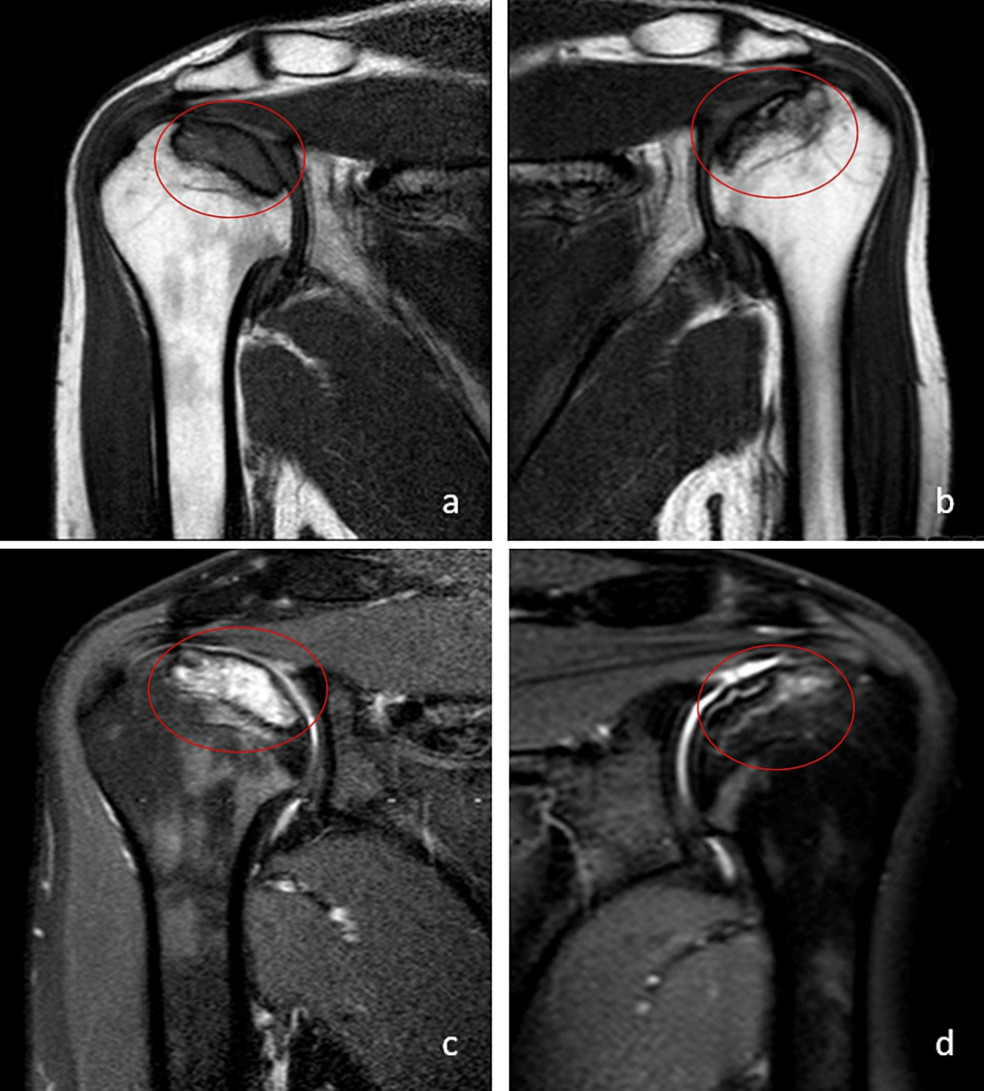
T1 and T2 sequence magnetic resonance images depicting avascular necrosis involvement of bilateral humeral heads. T1 sequence in the proximal right humerus (a) and in the proximal left humerus (b); T2 sequence in the proximal right humerus (c) and in the proximal left humerus (d). The lesions are marked with a red ellipse.
